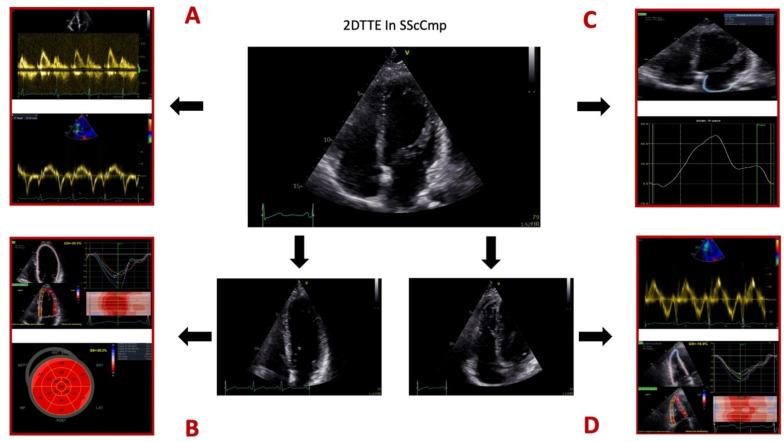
Different 2D transthoracic echocardiographic techniques used for evaluating the patients with systemic sclerosis and primary myocardial disease. Legend: Panel (A), mitral valve inflow profile by pulsed-wave (PW) Doppler, Tissue Doppler Imaging (TDI) for myocardial velocities. Panel (B), speckle-tracking echocardiography (STE) for assessing global longitudinal strain of the left ventricle (LV GLS). Panel (C), STE for assessing left atrial (LA) longitudinal strain. Panel (D), right ventricular (RV) evaluation comprised of TDI evaluation of RV free wall systolic velocity and RV free wall longitudinal strain. Images from the personal collection of the authors.
